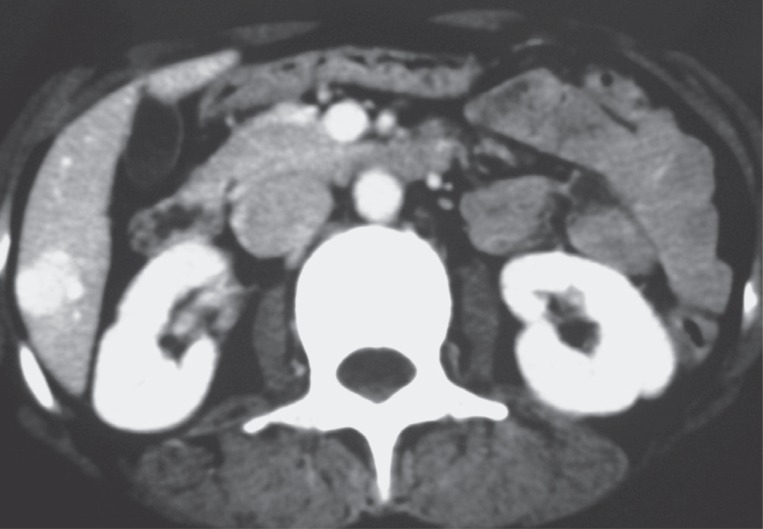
Axial CT scan after contrast injection in portal phase. The hepatic hemangioma has the same enhancement than that of the aorta.
